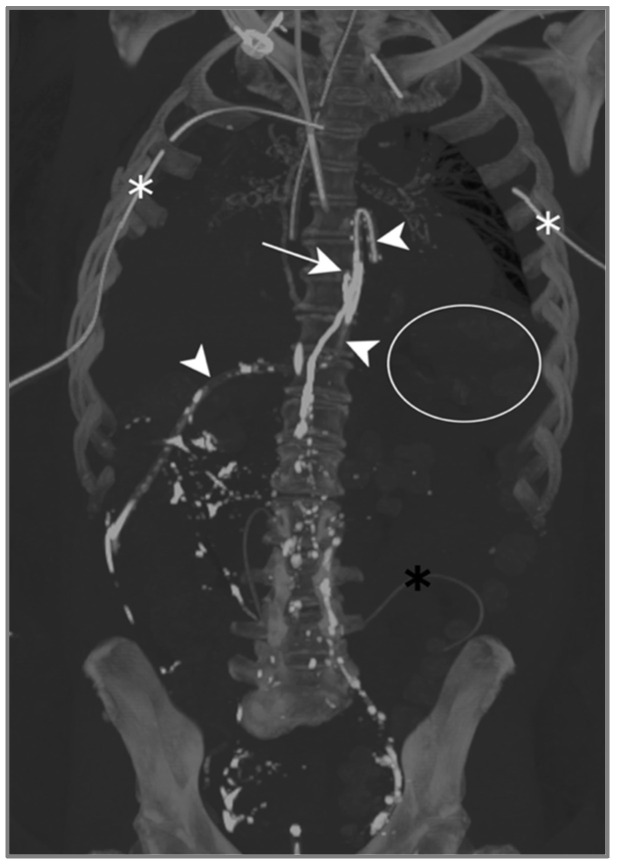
Patient A. The maximal-intensity projection of the CT scan reconstructed in the coronal plane. The Lipiodol injection shows opacification of the left inguinal and prevertebral lymphatic chains up to the level of the inferior vertebral plateau of D7 (white arrow). The drainage of the contrast agent through the catheter along the oesophagojejunal anastomosis is highlighted (white arrowheads). The subtle soft attenuation in the projection of the left lung field (white circle) corresponds to the accumulation of contrast in the pleural space due to the lymphatic rupture. The bilateral pleural catheter (white asterisks) and peritoneal drain (black asterisk) are identified.
